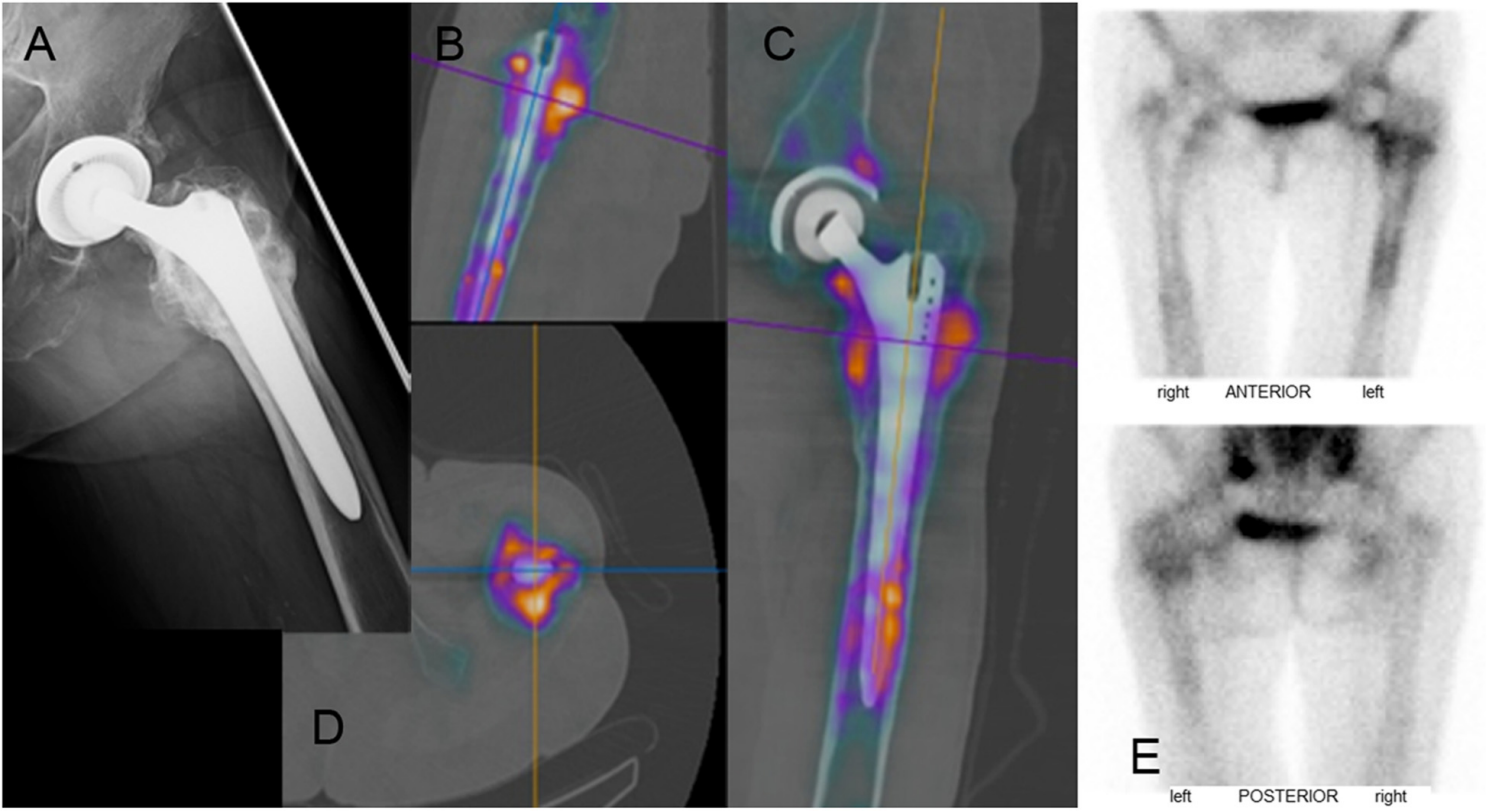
Example of pathological tracer uptake around a loosened stem. 80-year old female patient with THA of the left hip with a diaphyseal locking revision-stem. The patient had a history of several operations after a subtrochanteric femurfracture and complains now of therapy-resistant thigh-pain. A) shows the axial view of the left hip without definite signs of loosening. B)-D) shows pathological tracer uptake at the whole bone-prosthesis interface, consistent with the grading F3,1. The acetabular cup seen in C) shows isolated enhancement in the superior third, which was interpreted as physiological bone remodelling and graded A2 according to our classification. E) shows the anterior and posterior view of planar SPECT images of the pelvis. Intraoperative findings confirmed the diagnosis and the stem was exchanged.
